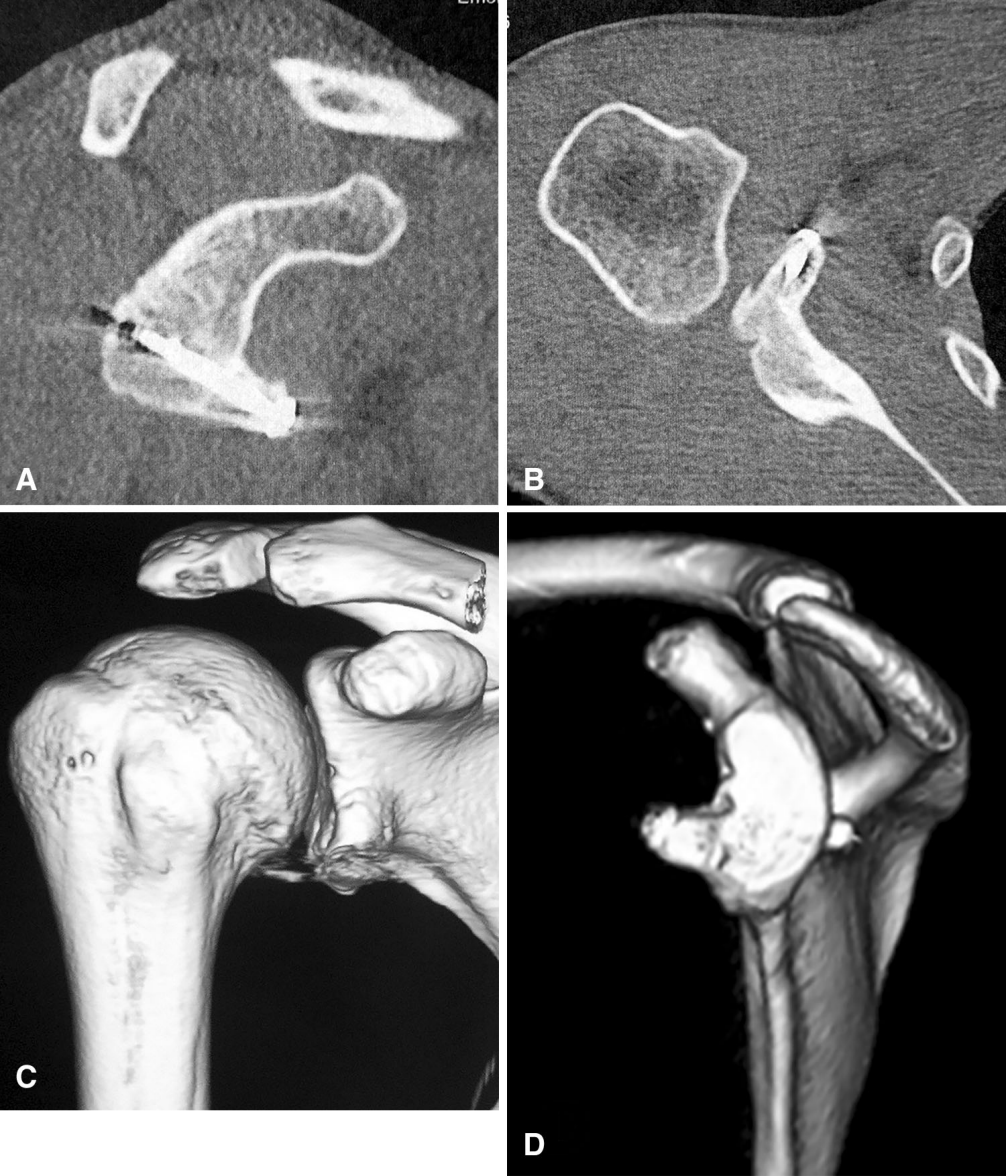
Postoperative (A) vertical and (B) axial CT scans and (C, D) three-dimensional CT reconstructions of the transferred coracoid bone graft demonstrate coracoid graft optimal positioning and healing.
